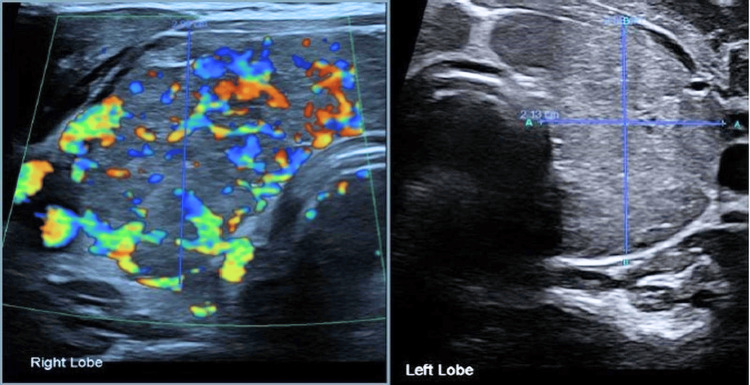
Thyroid ultrasound revealed diffusely enlarged and heterogeneous thyroid with markedly increased vascularity
